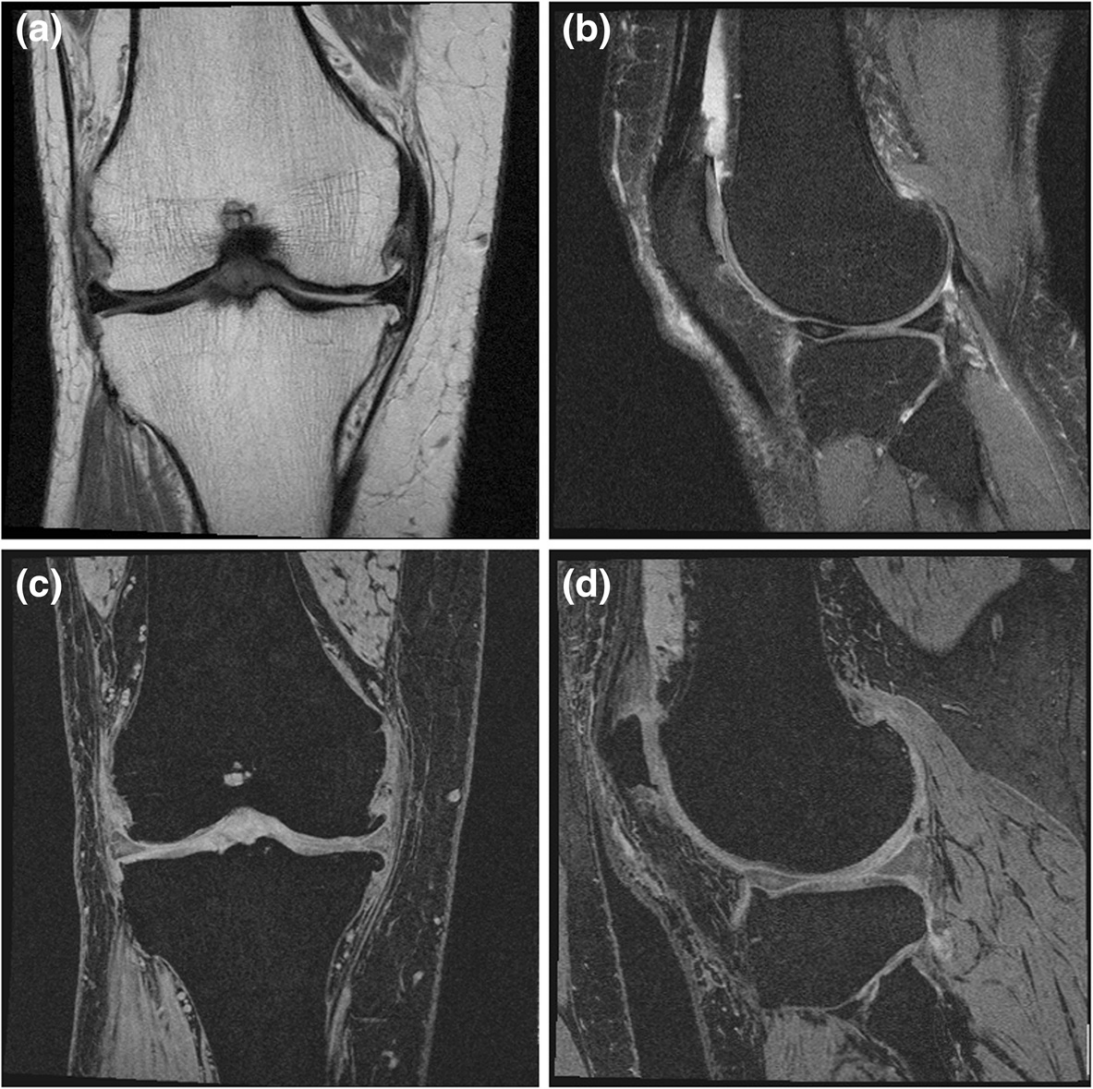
Examples of MR images; (a) 2D coronal TSE (TR/TE=3850/29 ms), (b) 2D sagittal TSE (TR/TE=3200/30 ms), (c) 3D coronal T1-weighted water-excitation FLASH (TR/TE=20/7.57 ms, flip angle=12 degrees), and (d) 3D sagittal water-excitation DESS (TR/TE=16.3/4.7 ms, flip angle =25 degrees) with coronal reformat, respectively.
